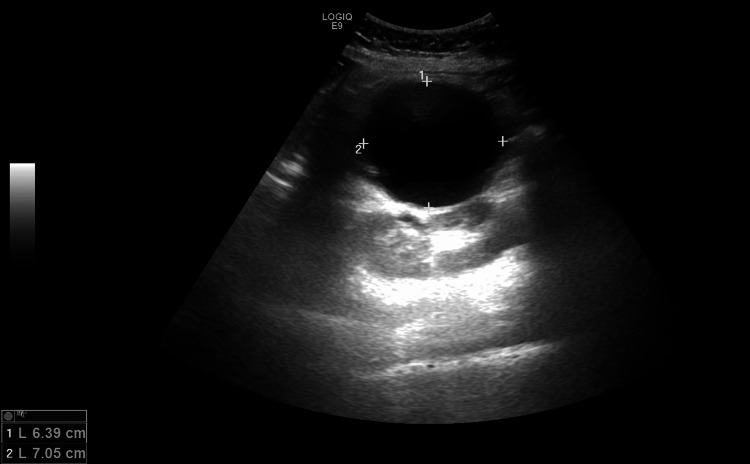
An abdominal ultrasound demonstrating a 7 x 6.4 cm well-defined anechoic cyst in the region of the pancreatic tail.
